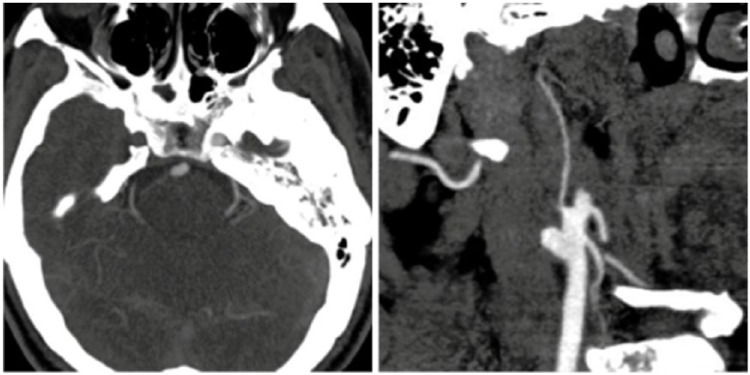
Computed tomography angiography shows loss of enhancement in the right
